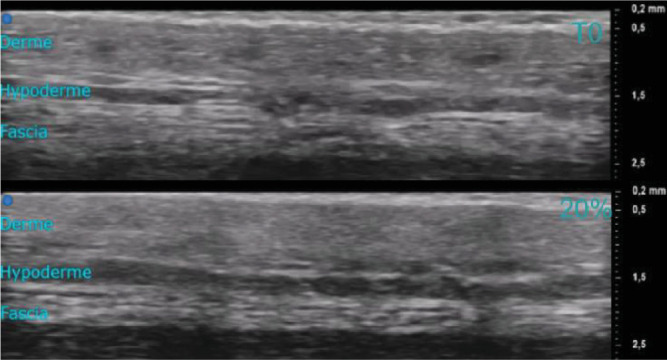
Ultrasound images of the skin during an extension test performed at the University Hospital of Saint-Etienne. The three deep layers of the skin can be seen: dermis, hypodermis and even the fascia at *t*0 and 20% extension. These images correspond to the forearm (*n* = 1 and man older than 30 years) following the same approach as for the relaxation tests (see protocol given in §2.2).
